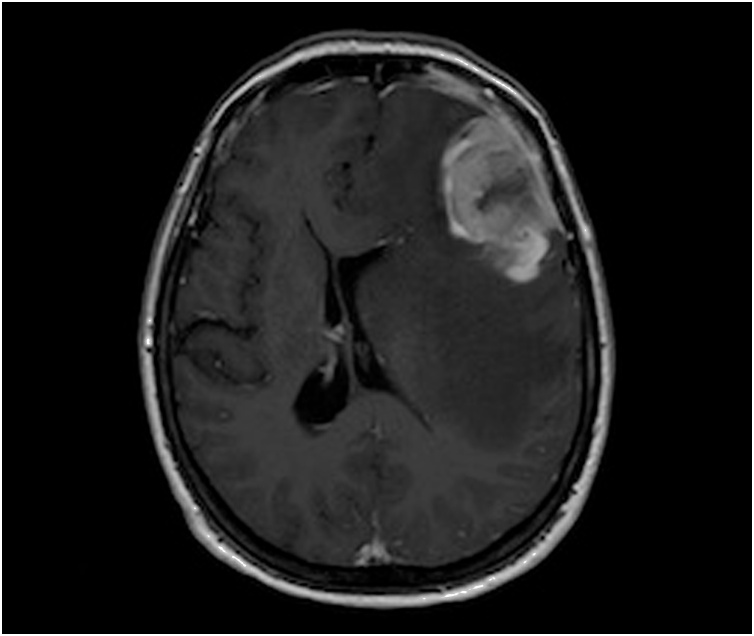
B-MRI shows a single extraxial left frontotemporal lesion of about 52 mm, which determined vasogenic edema and midline 11 mm shift.
